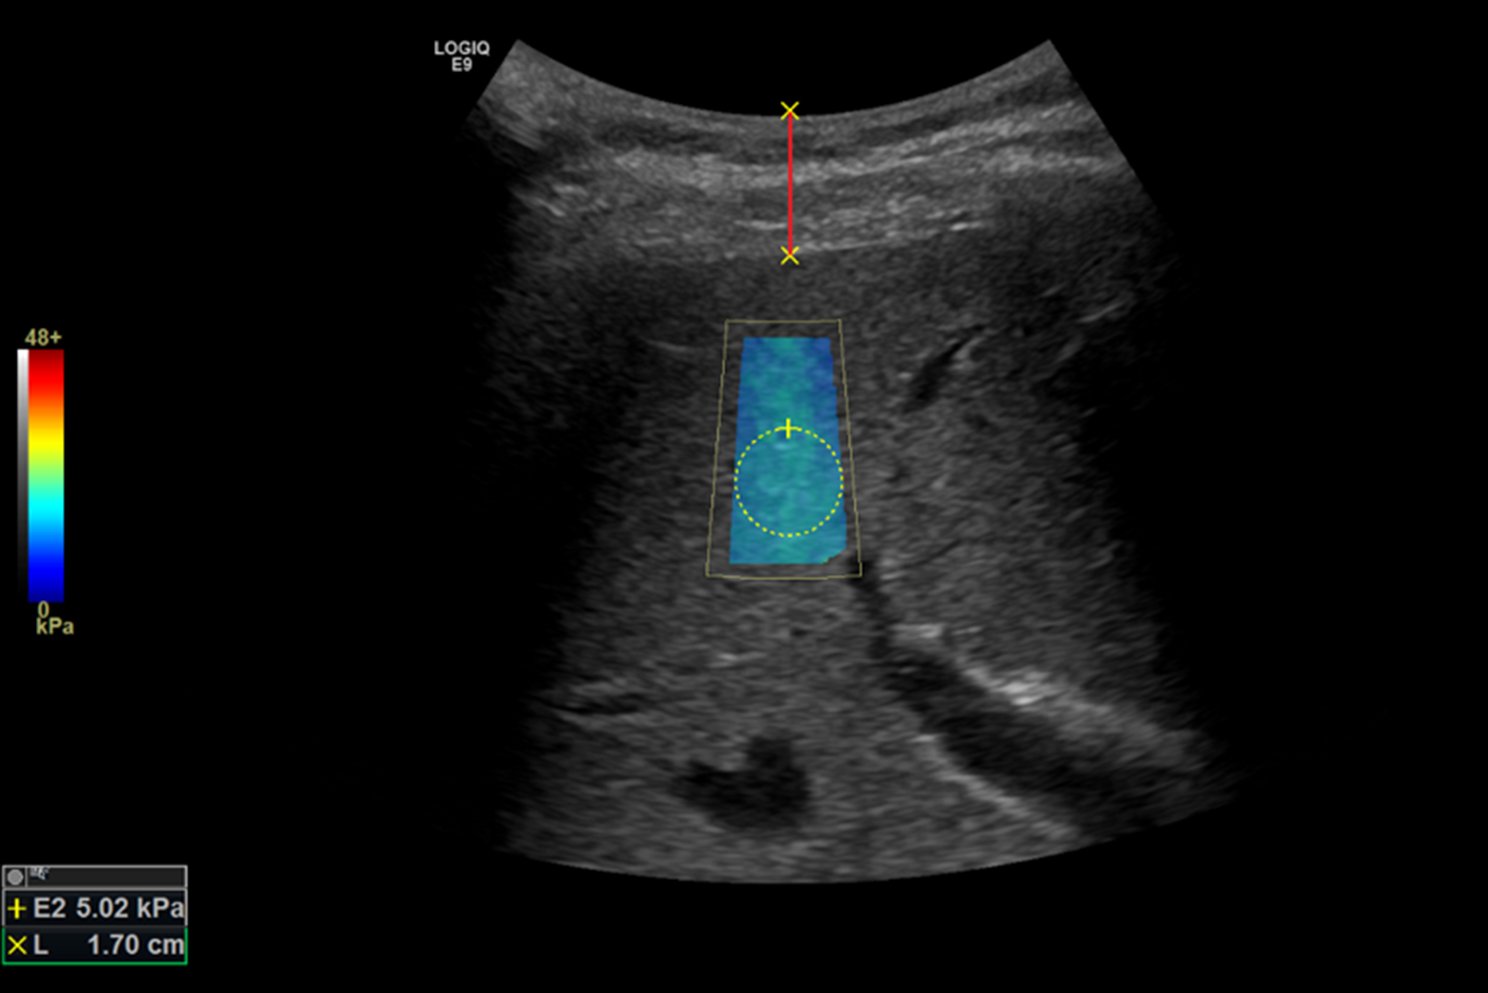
Ultrasound image: The fully color-filled shear wave elastography (SWE) and the homogeneous elastogram sample box indicate technical success and high-quality shear wave acquisition. The circle denotes the region of interest (ROI; spherical volume), while the red line represents the skin-to-liver capsule distance (SCD)
